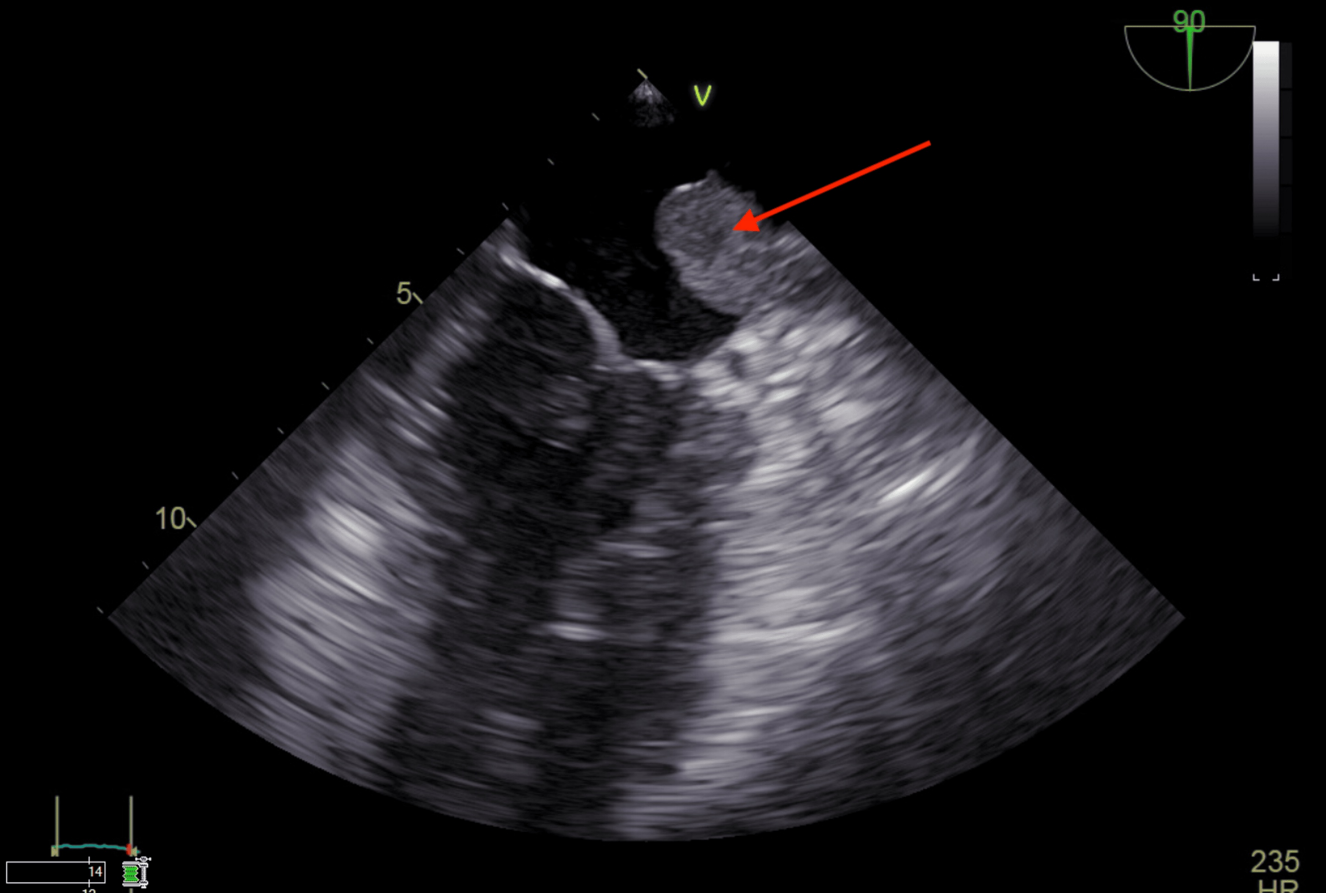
Transesophageal echocardiogram (TEE) showing an intracardiac thrombus inside the left atrium indicated by the red arrow
